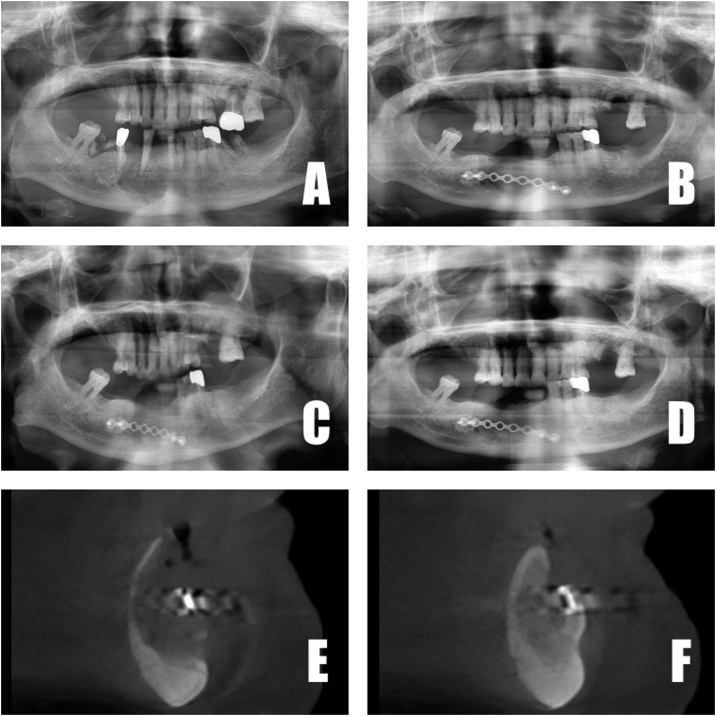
A. Panoramic X-ray at first visit. B. Panoramic X-ray 3 months postoperatively. E. Panoramic X-ray 6 months postoperatively. F. Panoramic X-ray 12 months postoperatively. G. CBCT after surgery. H. CBCT 6 months postoperatively.
